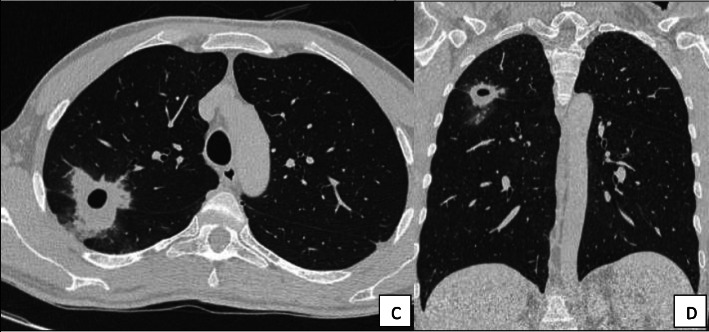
Involvement of the right upper and inferior lung lobe shown in chest CT in transverse (c) and coronal view (d)
